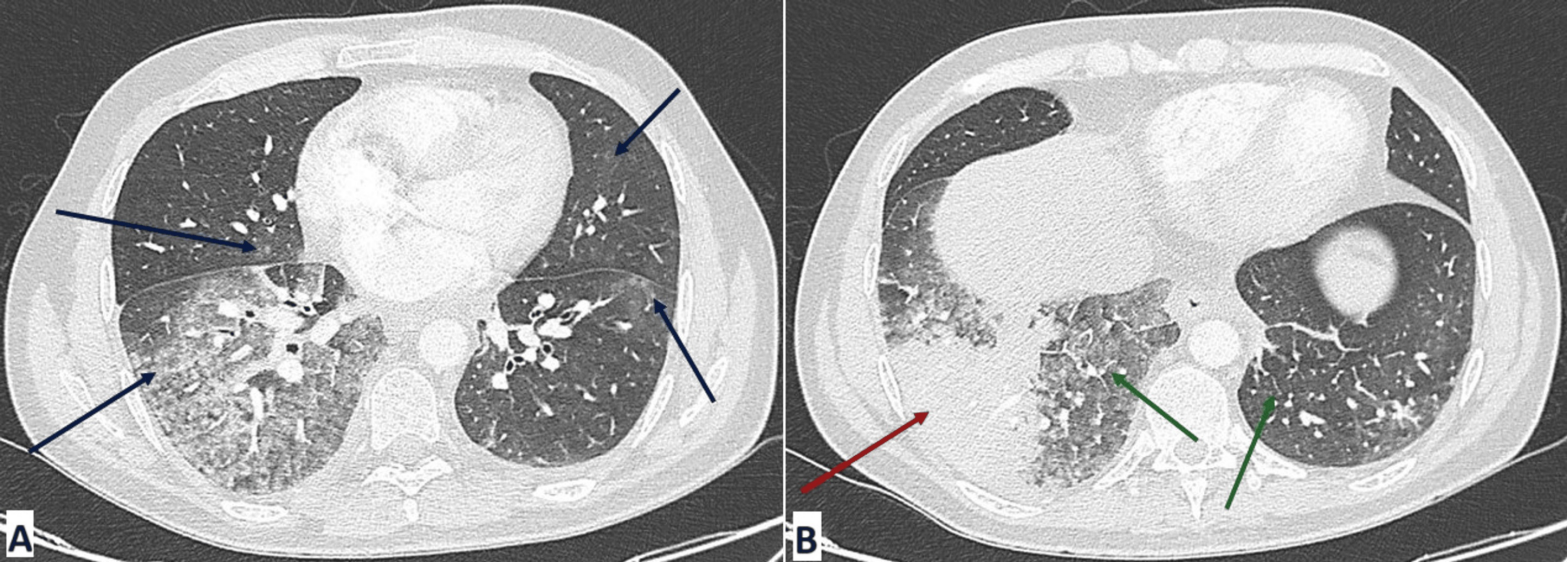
Chest CT scan on day 4 in lung window (A) Axial section at the mid-lung level showing diffuse ground-glass opacities (blue arrows) consistent with severe pneumonia. (B) Axial section at a lower lung level from the same scan, demonstrating consolidation in the right lower lobe (red arrow), and mild interlobular septal thickening (green arrows), further highlighting extensive bilateral lung involvement.
